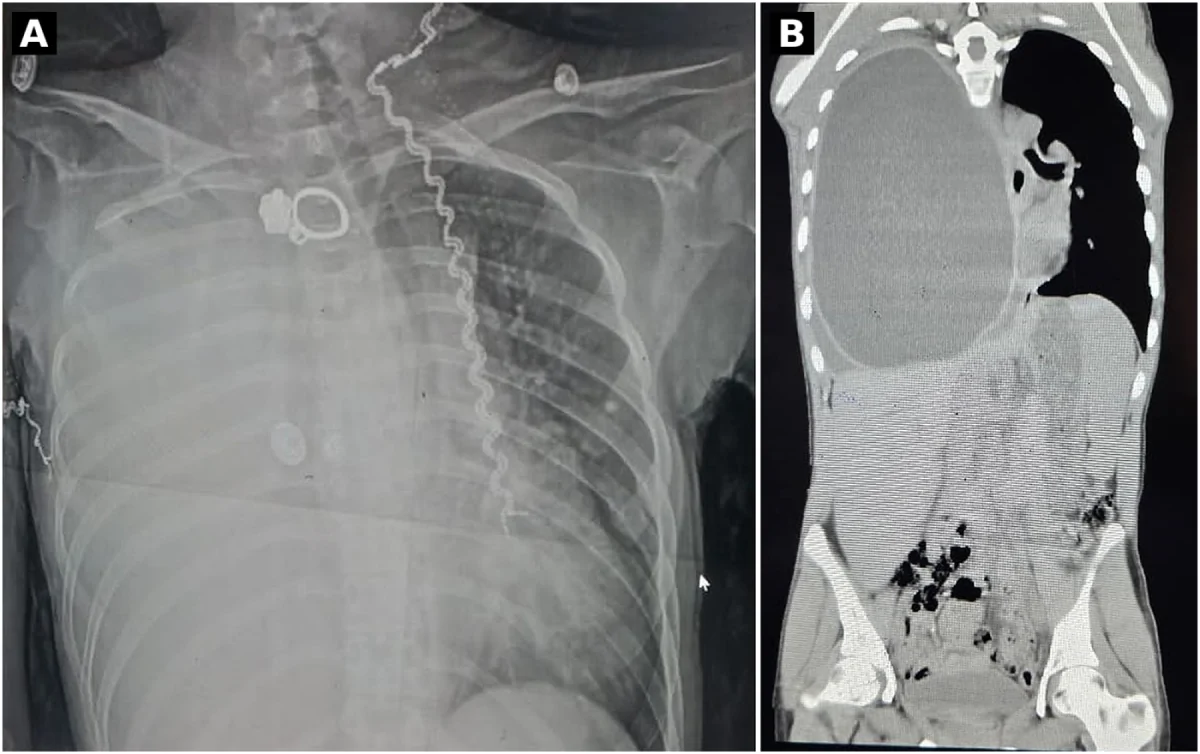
Preoperative radiological findings. (A) Frontal chest radiograph showing near-complete opacification of the right hemithorax with marked leftward displacement of the mediastinum and compression of the right lung. (B) Coronal contrast-enhanced computed tomography image demonstrating a single, well-defined, fluid-filled giant cyst occupying almost the entire right hemithorax, with severe compression of the residual right lung and substantial displacement of the mediastinum and heart to the left.
